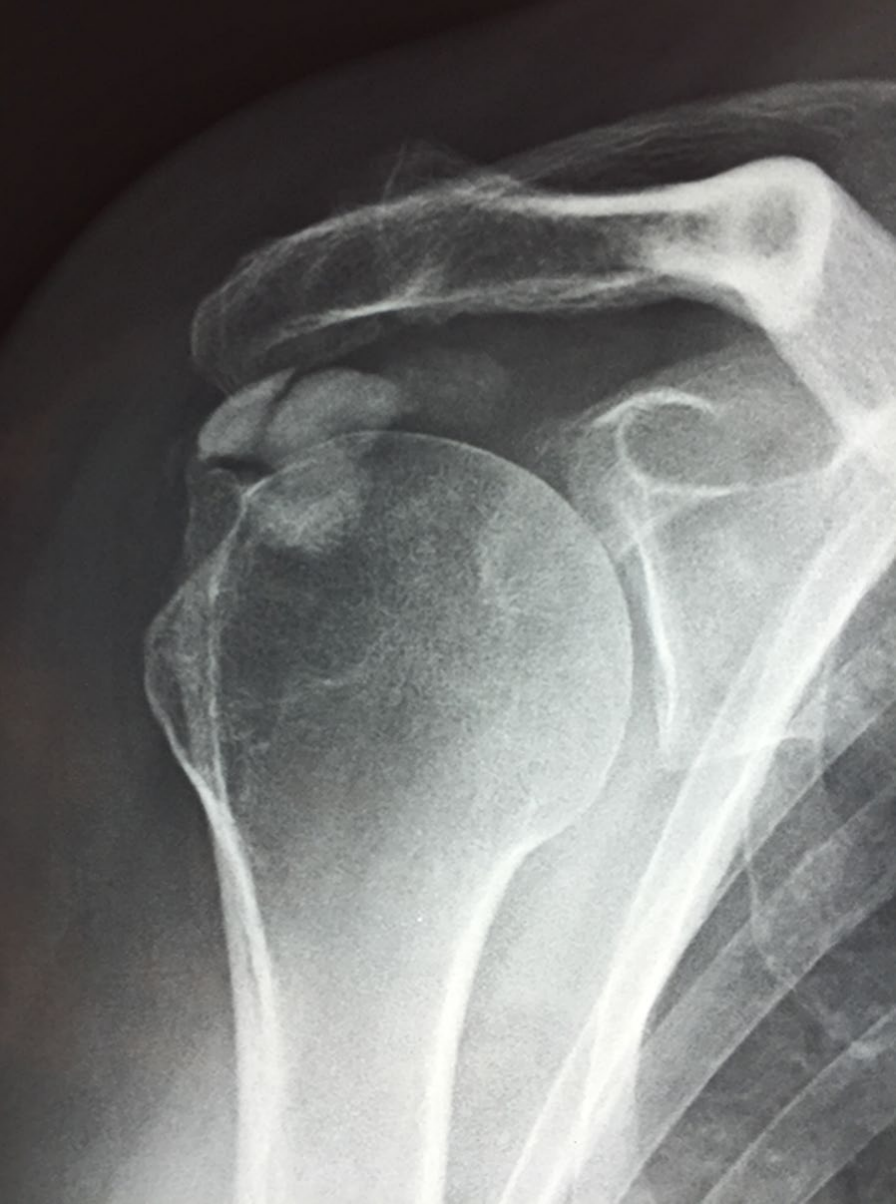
Residual calcification (Gärtner type 1). This patient was clinically and radiographically assessed 10  years after diagnosis. The x-ray showed the absence of arthritic signs
